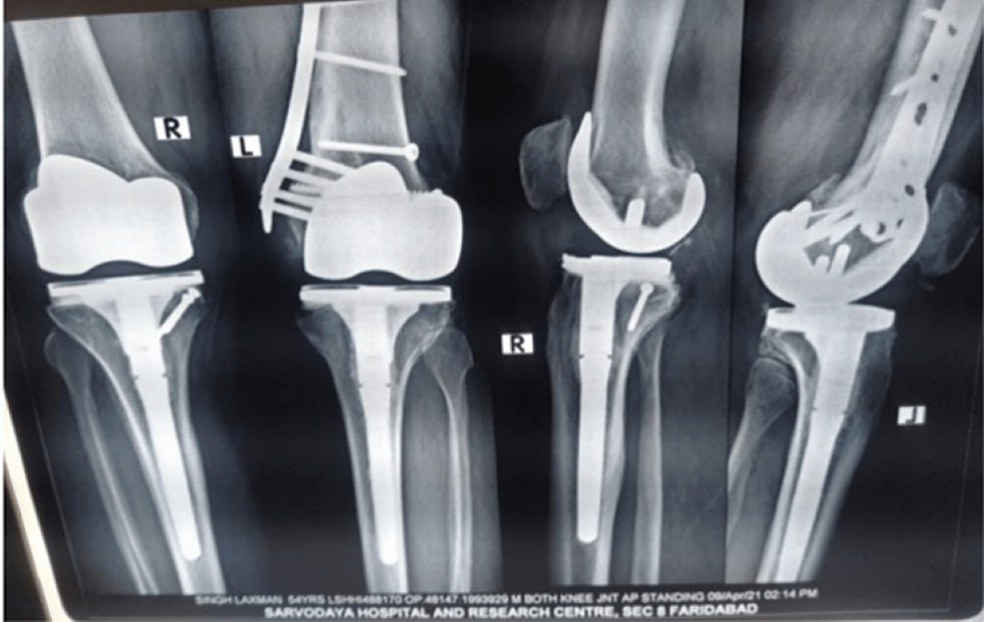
20-month follow-up X-rays showing the complete union of the fracture.
